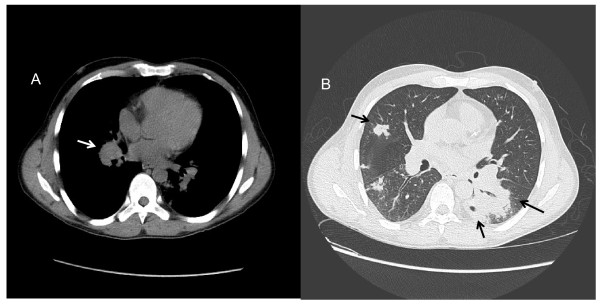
Thoracic CT Scan of case 2 at presentation. A: Right hilar lymphadenopathy. B: Multiple nodules in right lung. Areas of consolidation with cavitation in left lower lobe.
